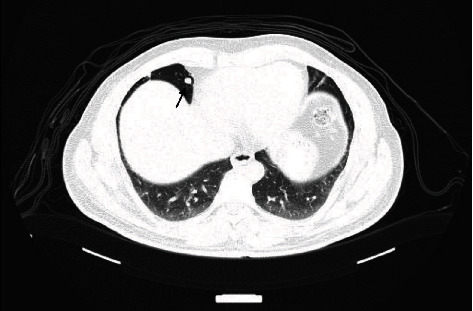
High-resolution chest CT shows a pulmonary nodule in the right middle lung lobe denoting pulmonary metastasis.
